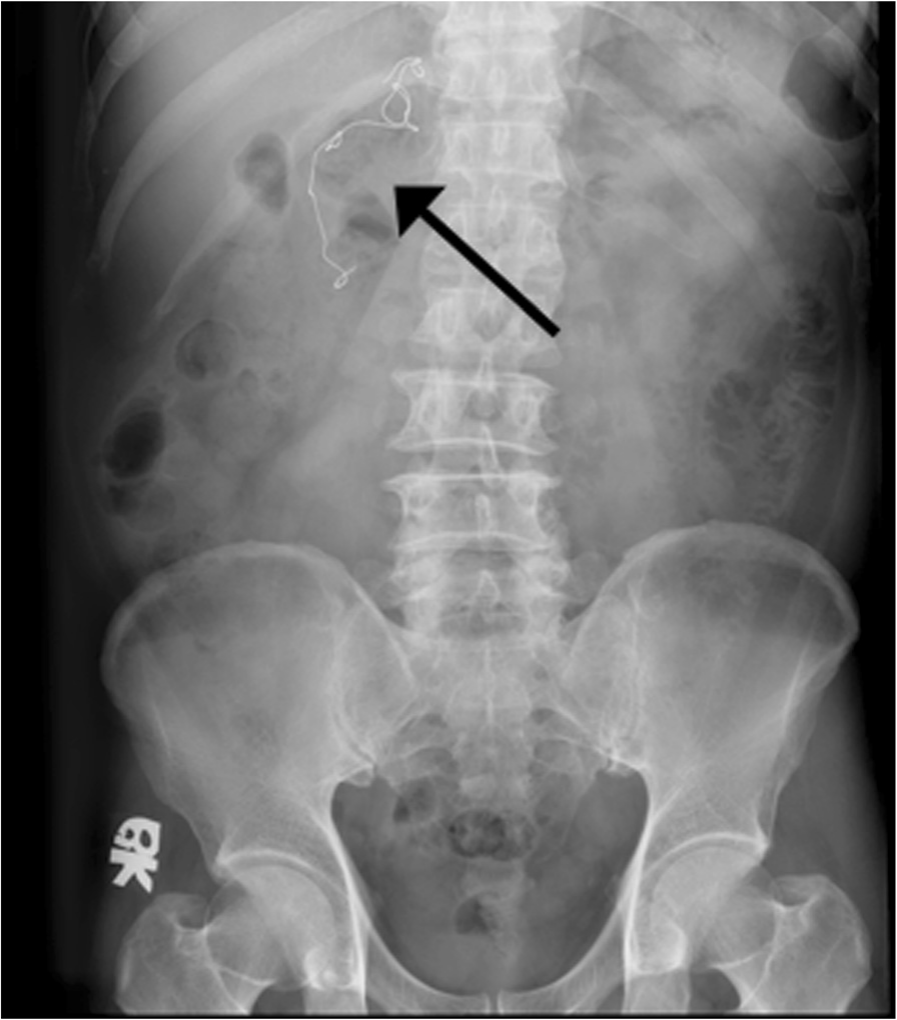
The plain film radiograph. Plain abdominal X-ray shows a retained surgical gauze (black arrow) in the abdominal cavity.
